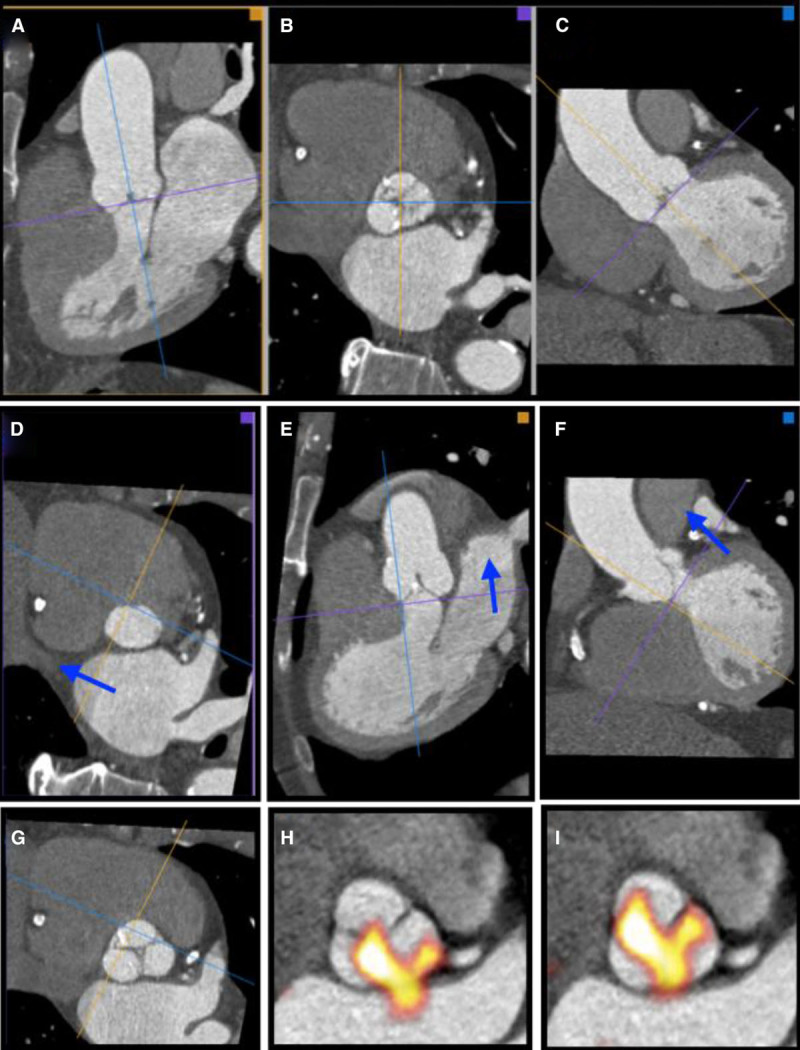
Creation of coregistered en face short-axis positron emission tomography (PET)/computed tomography (CT) images of the aortic valve. First, the CT angiogram is reorientated to get into the approximate plane of the aortic valve by lining up the axial cross hair (purple in this example) using the images in the coronal (A) and sagittal planes (C). This creates an approximate cross-sectional image of the aortic valve in the axial frame (B). Scrolling down in the axial frame, the center of the crosshairs is then placed over the exact point at which the right coronary cusp disappears, identifying the base of that leaflet (D). Similarly, the base of the noncoronary cusp is identified, and orthogonal planes adjusted so that the purple plane goes through the base of both these 2 cusps (D). Finally, the base of the left coronary cusp is found by rotation of the axial crosshairs so that first the cusp comes into view. The image is then slowly rotated in the opposite direction until the point where the leaflet first disappears (the base) is again found (F). This produces an en face image of the valve aligned with the base of all 3 leaflets (G). Adjacent 3-mm slices are then created in that plane and used for subsequent assessment. These slices are fused with the 18F-fluoride PET images (H) and careful coregistration performed in 3 dimensions to ensure accurate alignment between the PET and CT images (I).
